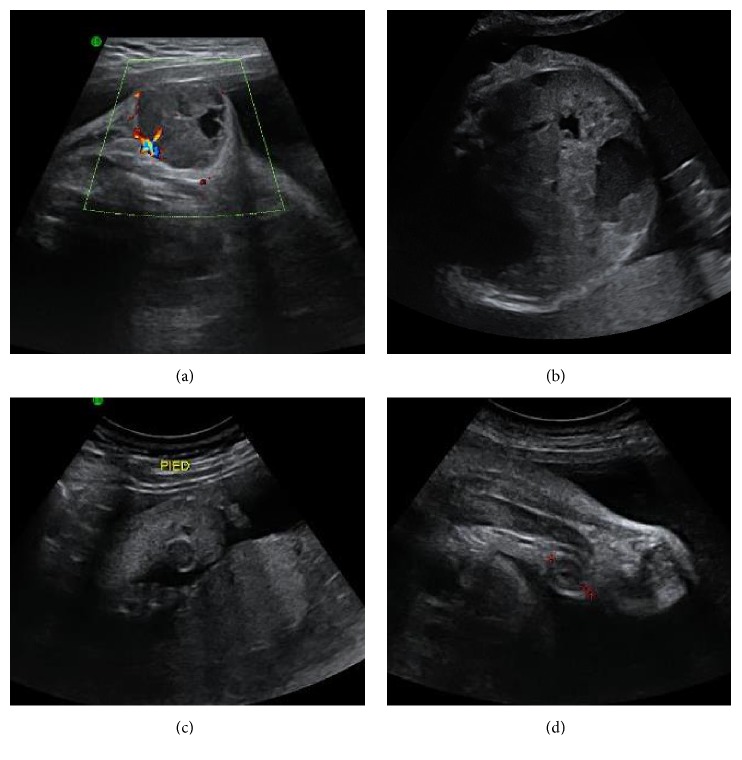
Other tumor sites on the iliac bone (a), intra-abdominal organs (b), left foot (c), and left thigh (d) found by ultrasound examination.
